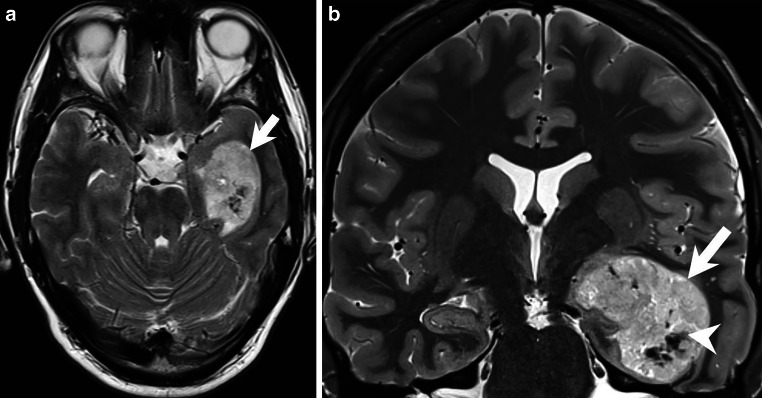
Axial (a) and coronal (b) T2-weighted images showing a clearly demarcated, intra-axial and space-occupying lesion in the left temporal lobe (*arrows*). The lesion had a relatively homogeneous hyperintense signal with circumscribed areas of signal loss in the lower parts of the tumor (b, *arrowhead*)
